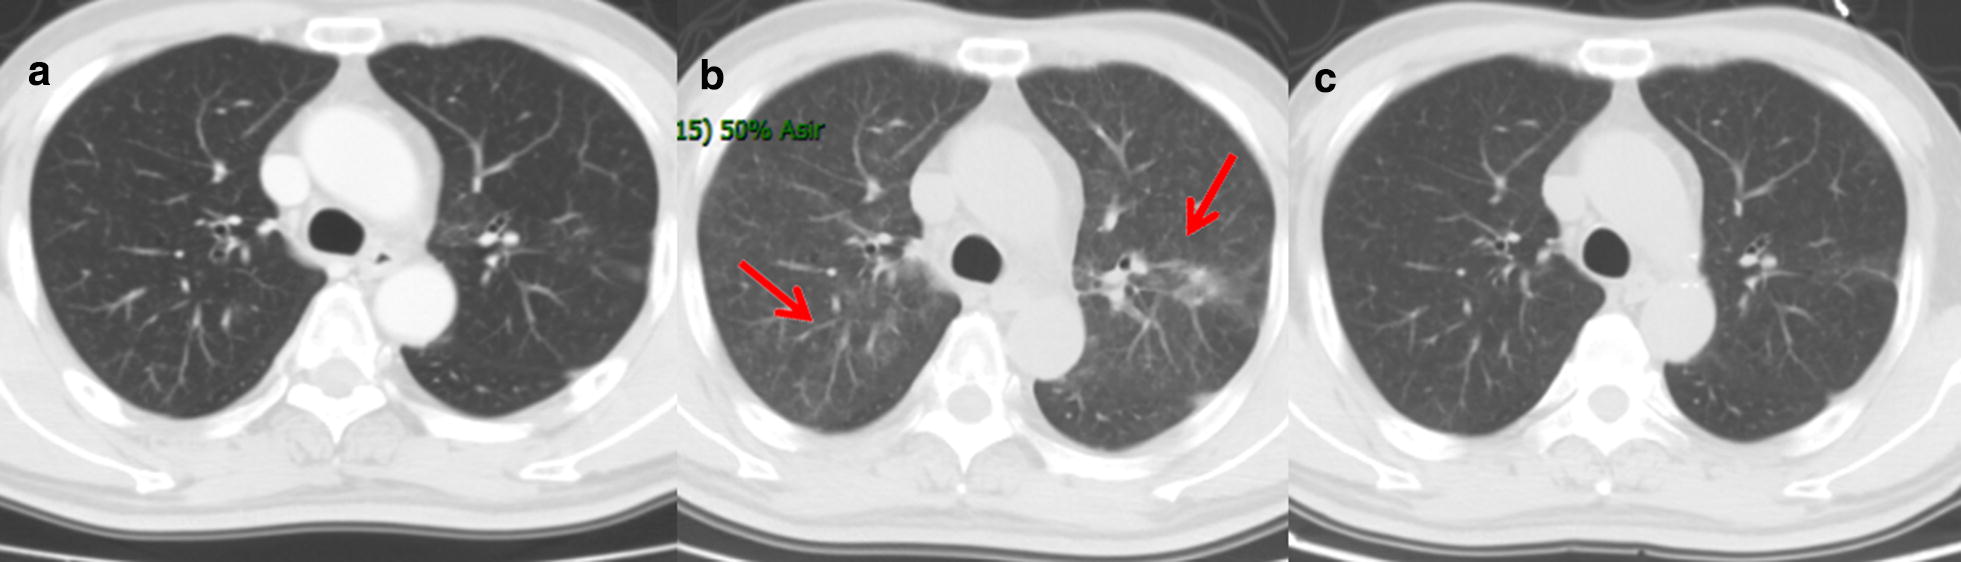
The radiographic findings of one patient with radiation pneumonitis complicated by *Pneumocystis carinii* for three courses. a The CT image acquired before irradiation therapy. b The CT image acquired when RP complicated by PCP was diagnosed. c The CT image acquired after TMP-SMX treatment. *RP* radiation pneumonitis, *PCP Pneumocystis carinii* pneumonia, *TMP–SMX* trimethoprim–sulfamethoxazole
